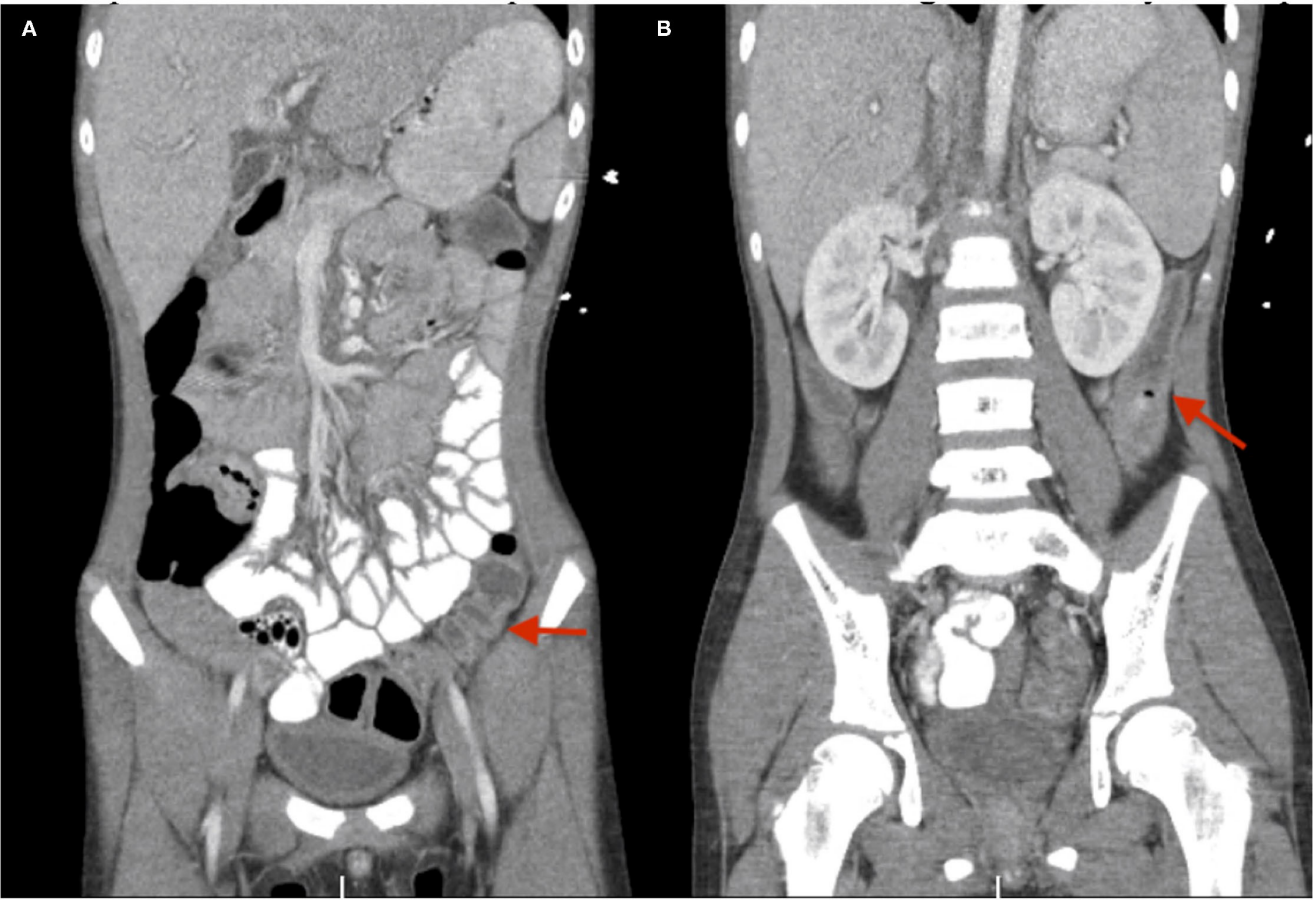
(A,B) CT abdomen pelvis with contrast for case #1. Red arrows depict the descending colon edema.
